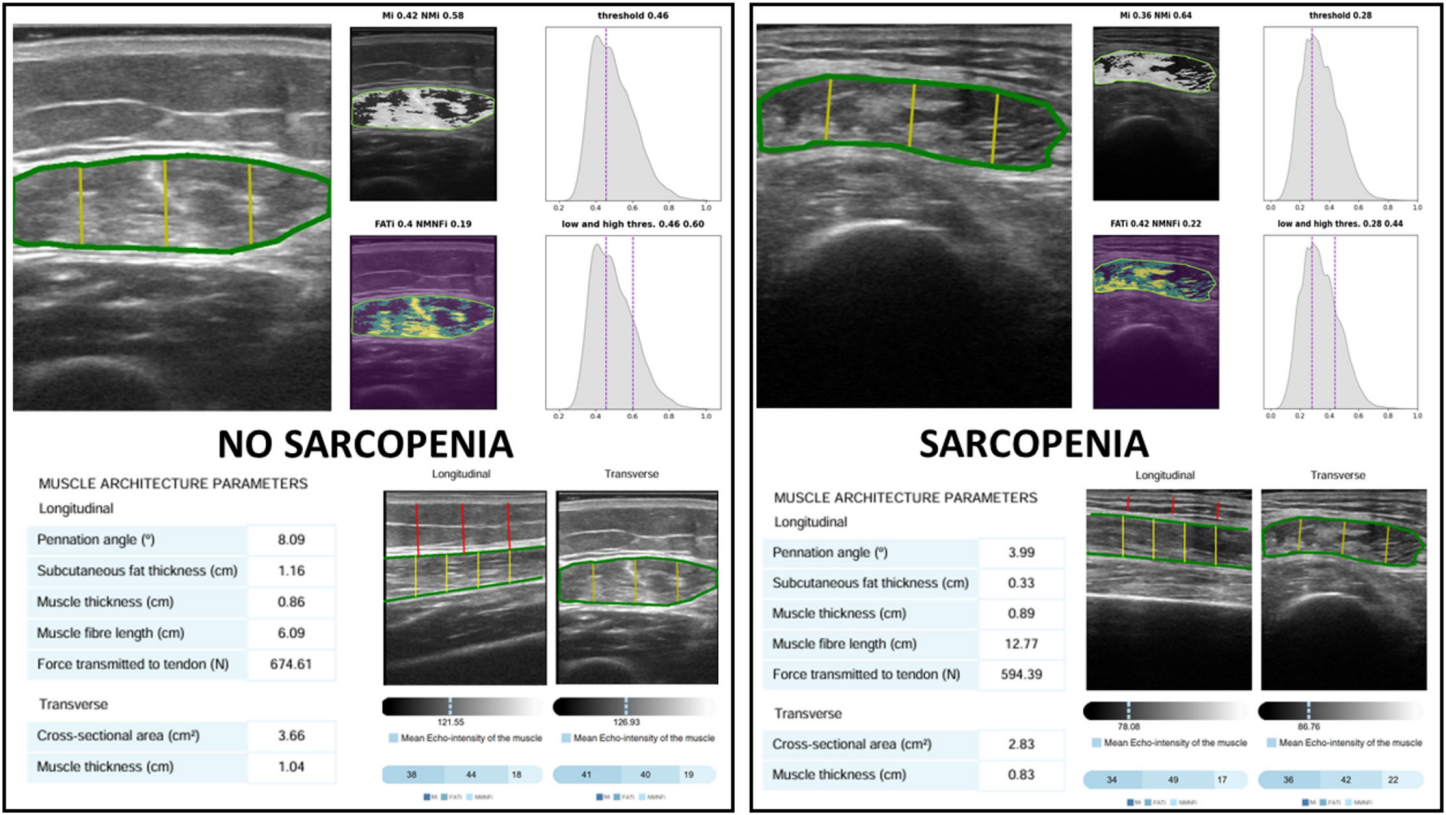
Image analysis by PIIXMED in a patient with sarcopenia and other without sarcopenia. Upper images: cross‐sectional quadriceps rectus femoris ultrasonography image from two patients: one diagnosed with sarcopenia and one without, based on EWGSOP2 criteria. The images were analysed using artificial intelligence, including histograms and thresholds derived from the multi‐Otsu algorithm. The pixels are differenced as low echogenicity (purple), medium echogenicity (green) and high echogenicity (yellow); Lower images: Output of the PIIXMED tool with numeric measures and the muscle echogenicity percentage of Region of Interest in the bottom bar low echogenicity (Mi), medium echogenicity (FATi), high echogenicity (NMNFi).
